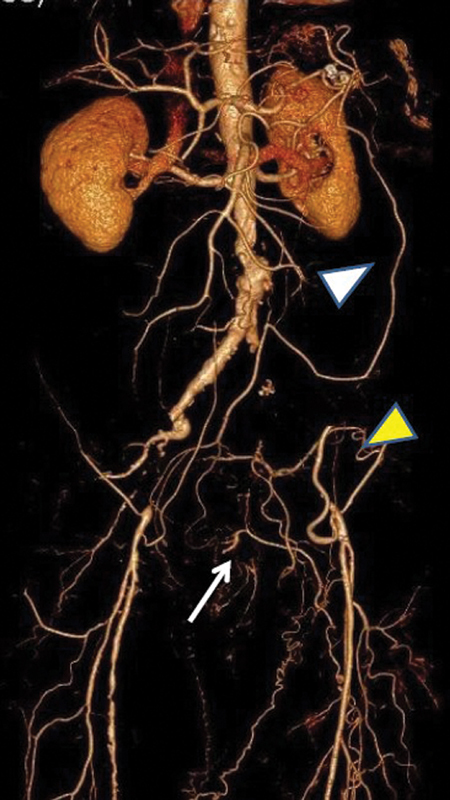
Computed tomography angiography of the patient with complete occlusion of the left common and external iliac artery and occlusion of the right external iliac artery. A complex network of systemic and visceral anastomotic vessels restores the flow to the lower limbs (for details see in the text).
